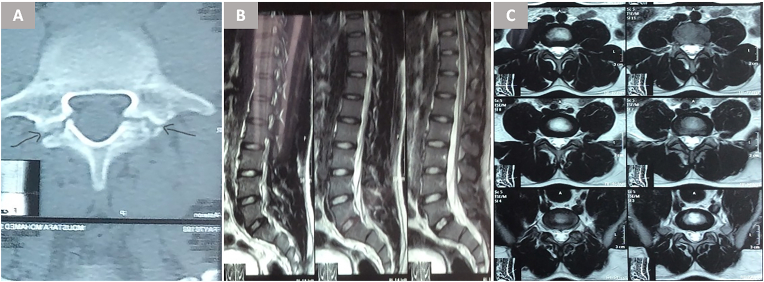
Preoperative imaging of a 25-year-old female with refractory low-back pain. (A) Axial CT shows bilateral L5 pars fractures without significant spondylolisthesis. (B, C) MRI excludes disc degeneration or spinal canal pathology.
